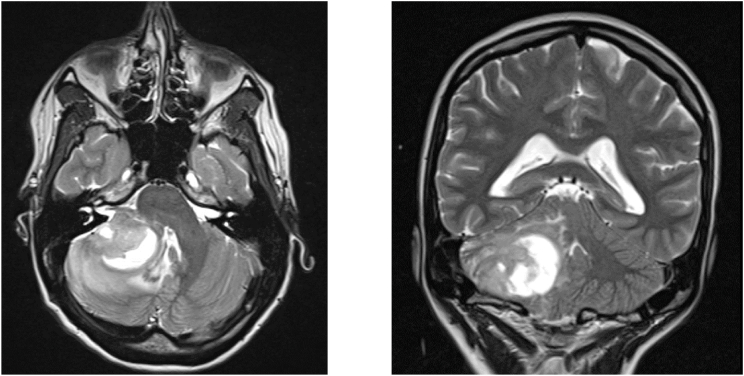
Brain MRI without contrast at admission, axial (left) and coronal (right) T2 sequence.
